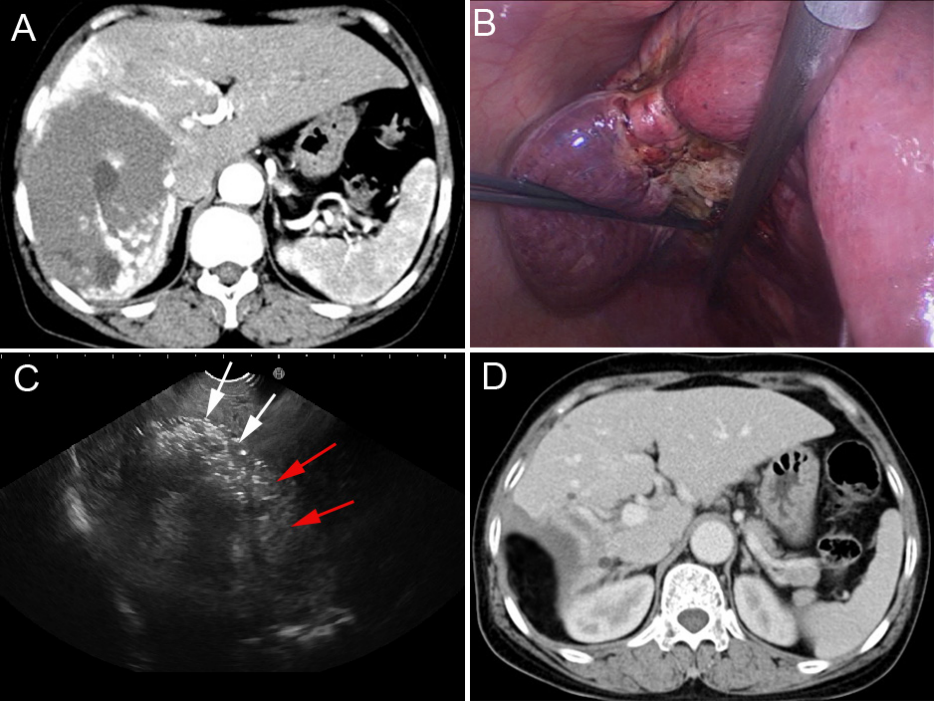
CT and intraoperative photos of case 2 A. Contrast-enhanced CT demonstrated a 13.1 cm hepatic hemangioma in the right lobe. B. Radiofrequency ablation induced the significant shrinking of the hemangioma along the resection margin. C. Intraoperative ultrasound imaging was used to determine the boundary (red arrows) of hepatic hemangioma (white arrows) in liver parenchyma. D. Contrast-enhanced CT shows that the hemangioma was completely resected without residual tissue.
